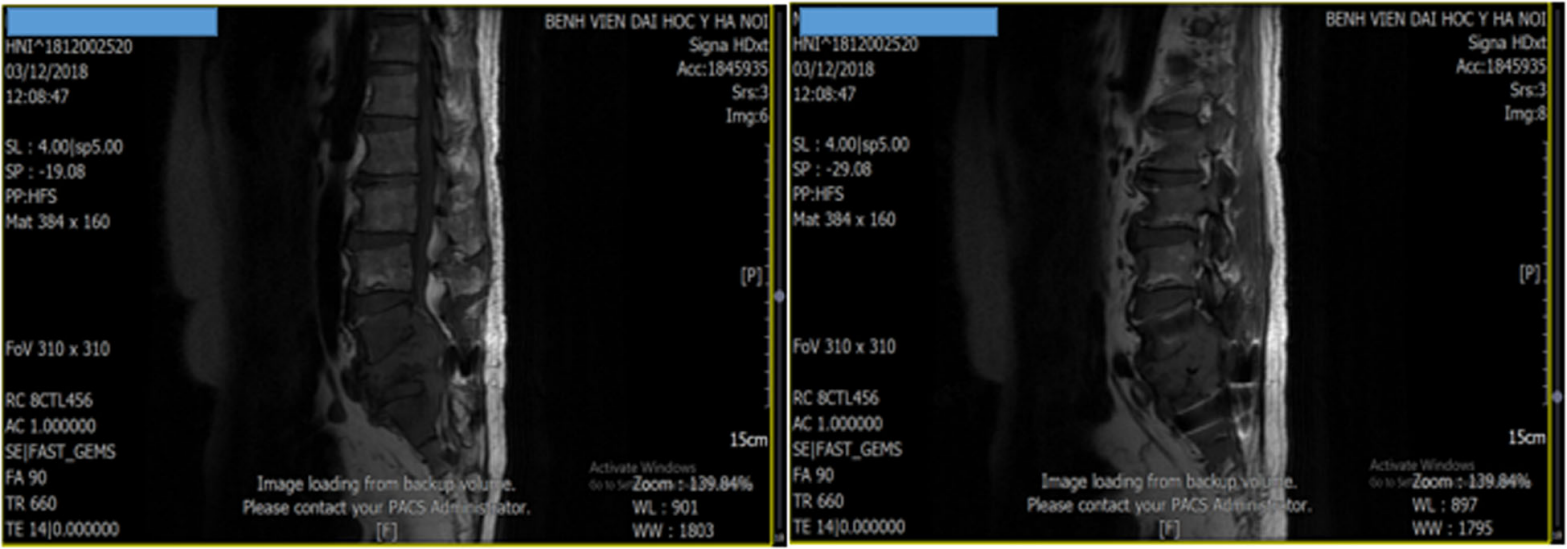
Lumbosacral spine magnetic resonance imaging revealed enlarged lytic lesions of S1 with ilium, soft tissue invasion, and compression of the nerve roots from L5 to S2 (December 2018)
